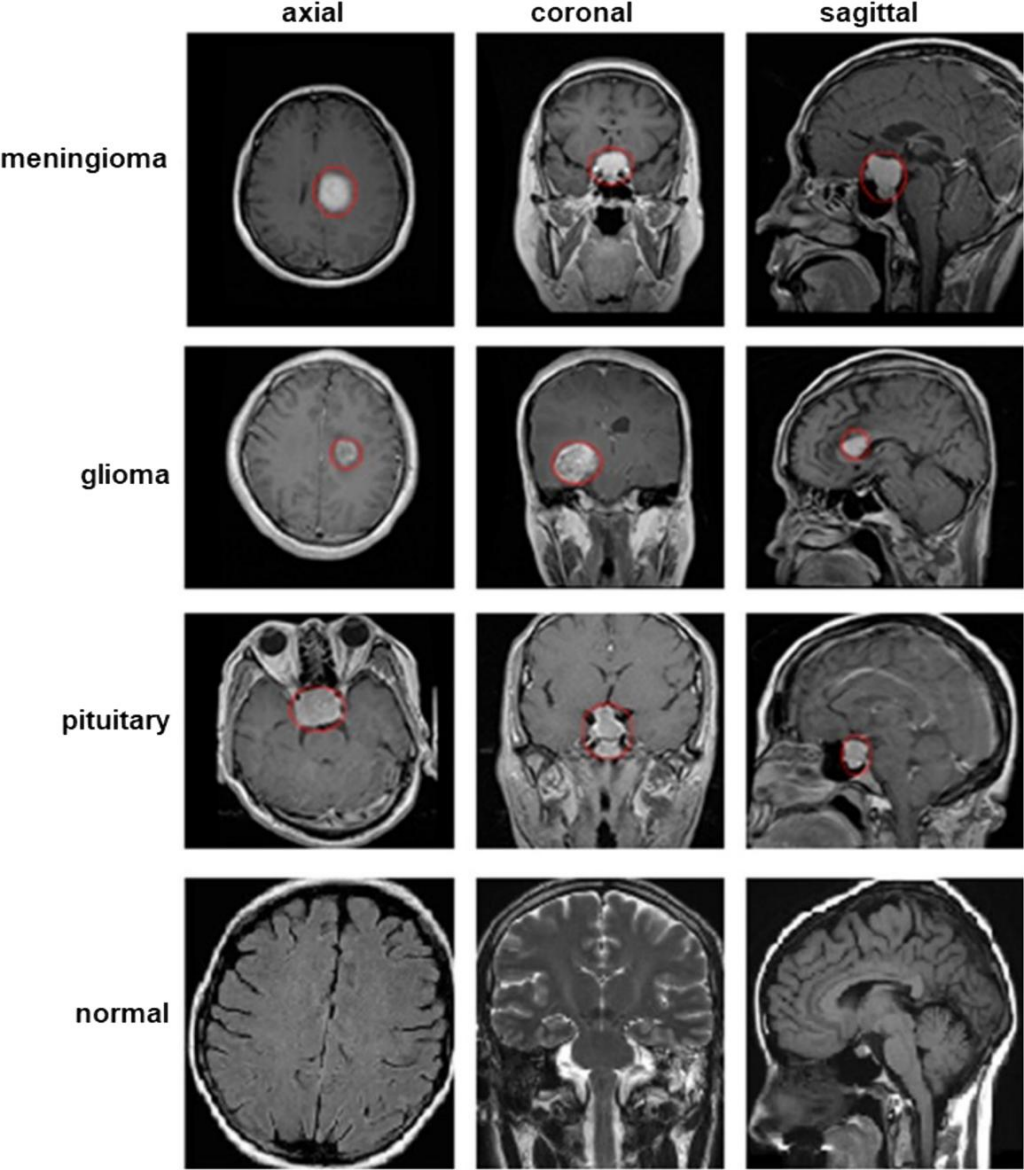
A class-labelled brain tumour dataset of a brain MRI image.
